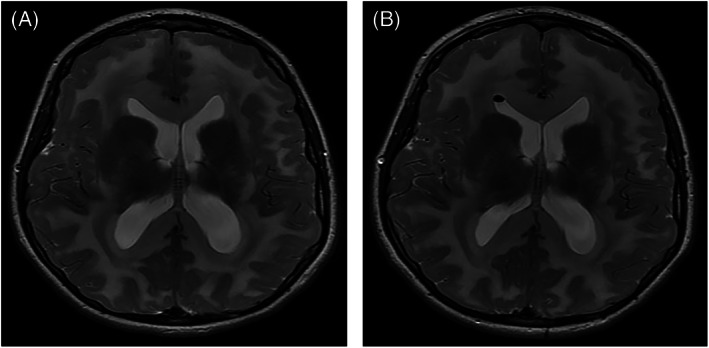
Axial T2 MRI of female patient diagnosed by HRS, aged 14 years demonstrates mild ventricular dilatation. Ventricles are larger than on baseline prior MRI studies. There is extensive hyperintensity of the white matter in keeping with GA type I (A). Axial T2 MRI obtained 1 week later (B) and 1 day post an Endoscopic Third Ventriculostomy (ETV) shows an interval slight decrease in ventricular size. There is a small bubble of air in the nondependent aspect of the right lateral ventricle.
